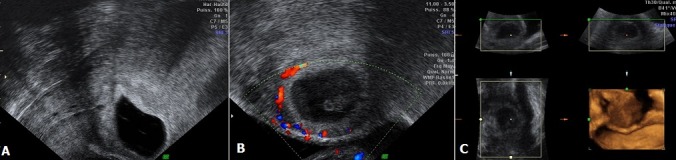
A) transvaginal echography in sagittal section showing a gestational sac implanted in the myometrium between the bladder and the anterior wall of the uterus with emptiness of the uterus and cervical canal; B) doppler ultrasound with color mode showing a periovulatory hyper-vascularization; C) ultrasound with 3D mode objectifying a gestational sac bulging through the anterior wall of the uterus into the bladder with diminished myometrial layer between the bladder and the sac
